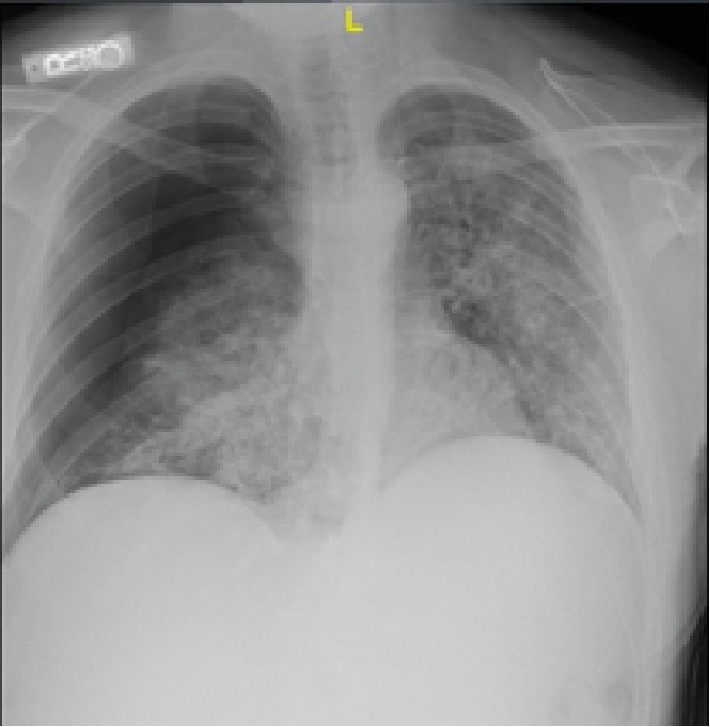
Chest X-ray showing bilateral parenchymal infiltrates and right-sided pneumothorax.
